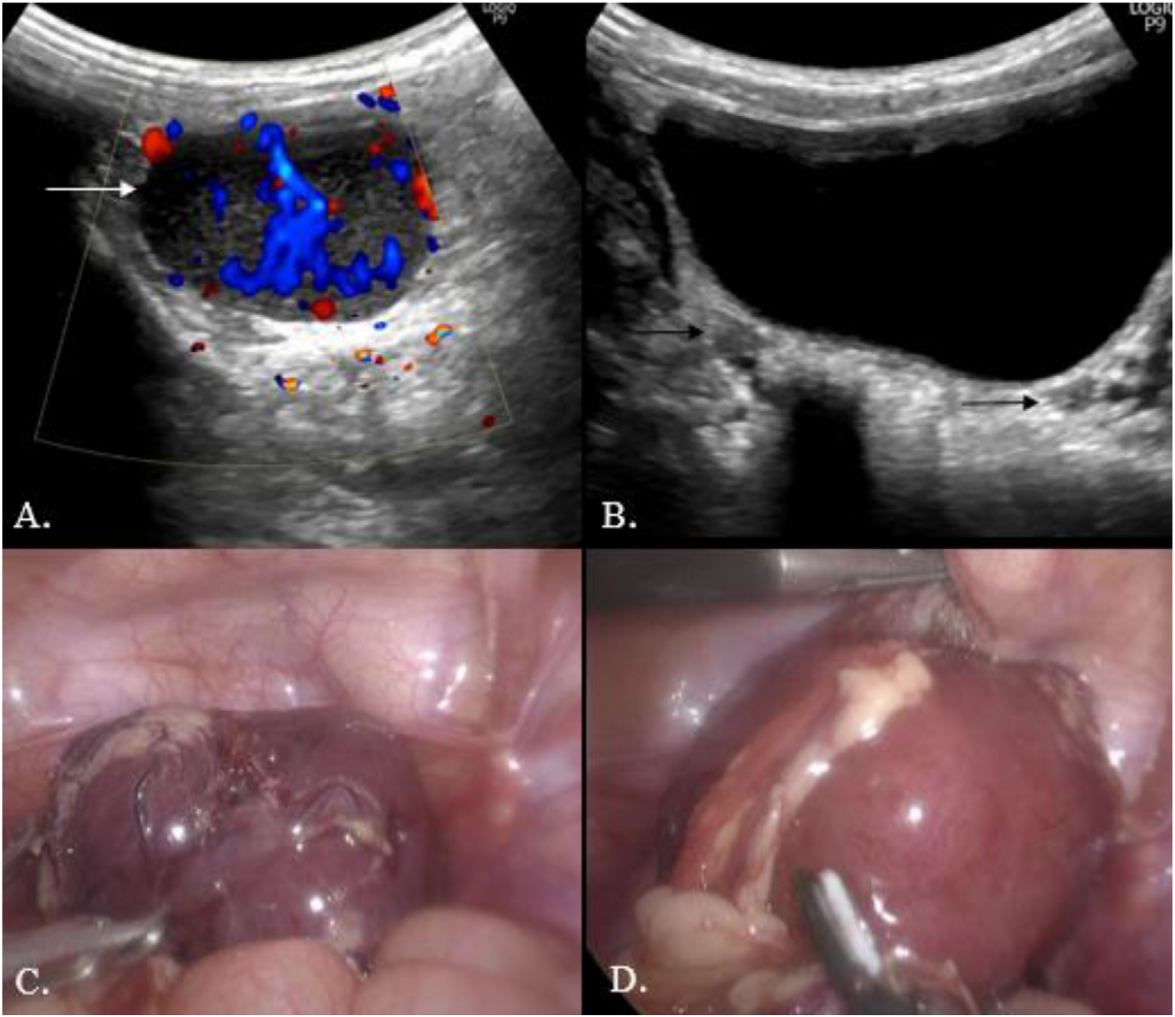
(A) The color Doppler image shows a well-defined hypoechoic lesion (white arrow) with significant internal vascularity. (B) Gray-scale image shows the right and left ovaries (black arrow) (C and D) Intraoperative images show a well-circumscribed lesion with a few vascular channels along the surface of the lesion.
